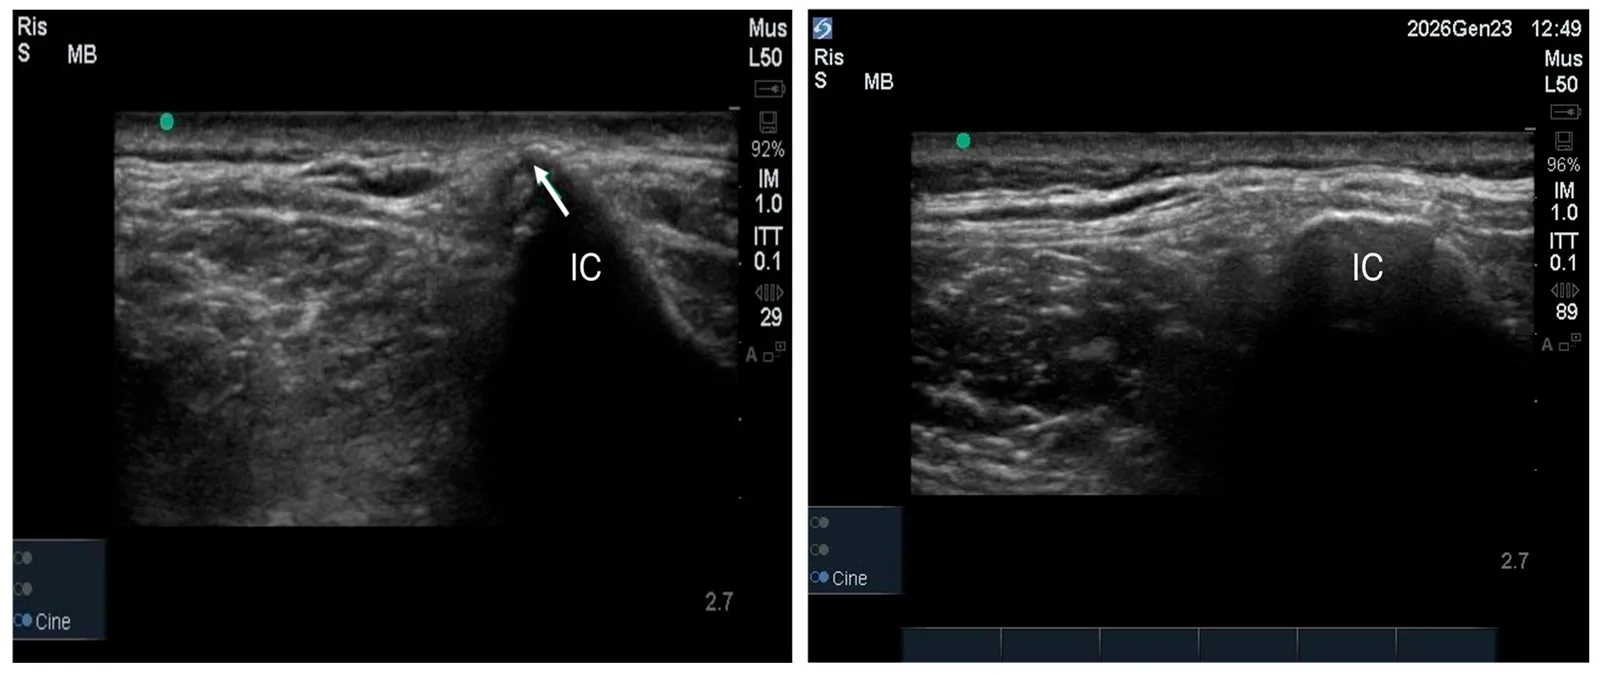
Ultrasound image of the iliac insertion site in short-axis view. The left side shows the presence of an iliac crest enthesophyte (white arrow) at the TLF insertion site; the right side shows a normal osseous profile of the iliac crest in a healthy control. IC: iliac crest.
